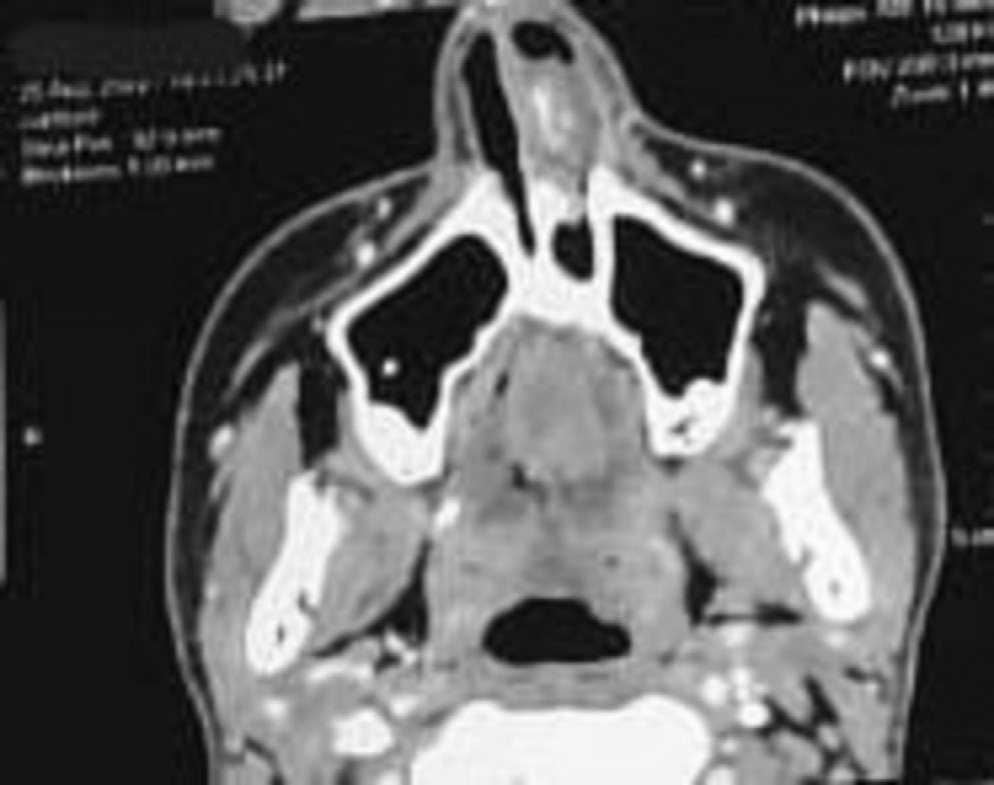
A nose and paranasal sinuses CT scan shows a mass in the left nasal cavity.
